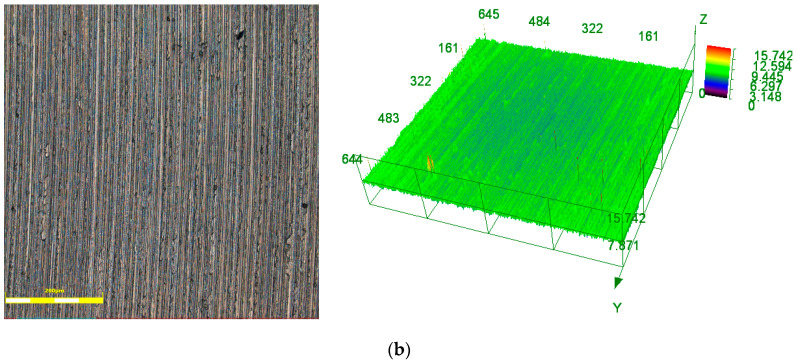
The microstructure of the ground surface (a) without ultrasonic vibration; (b) with ultrasonic vibration.
